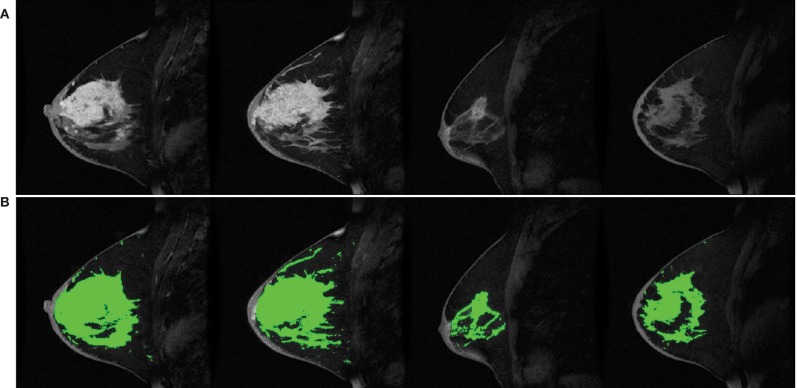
High-resolution 1.5T DCE MRI of four subjects from the American College of Radiology Imaging Network (ACRIN) 6657 repository (31) (A) unmodified and (B) with the segmented breast fibroglandular tissue overlaid in green.
